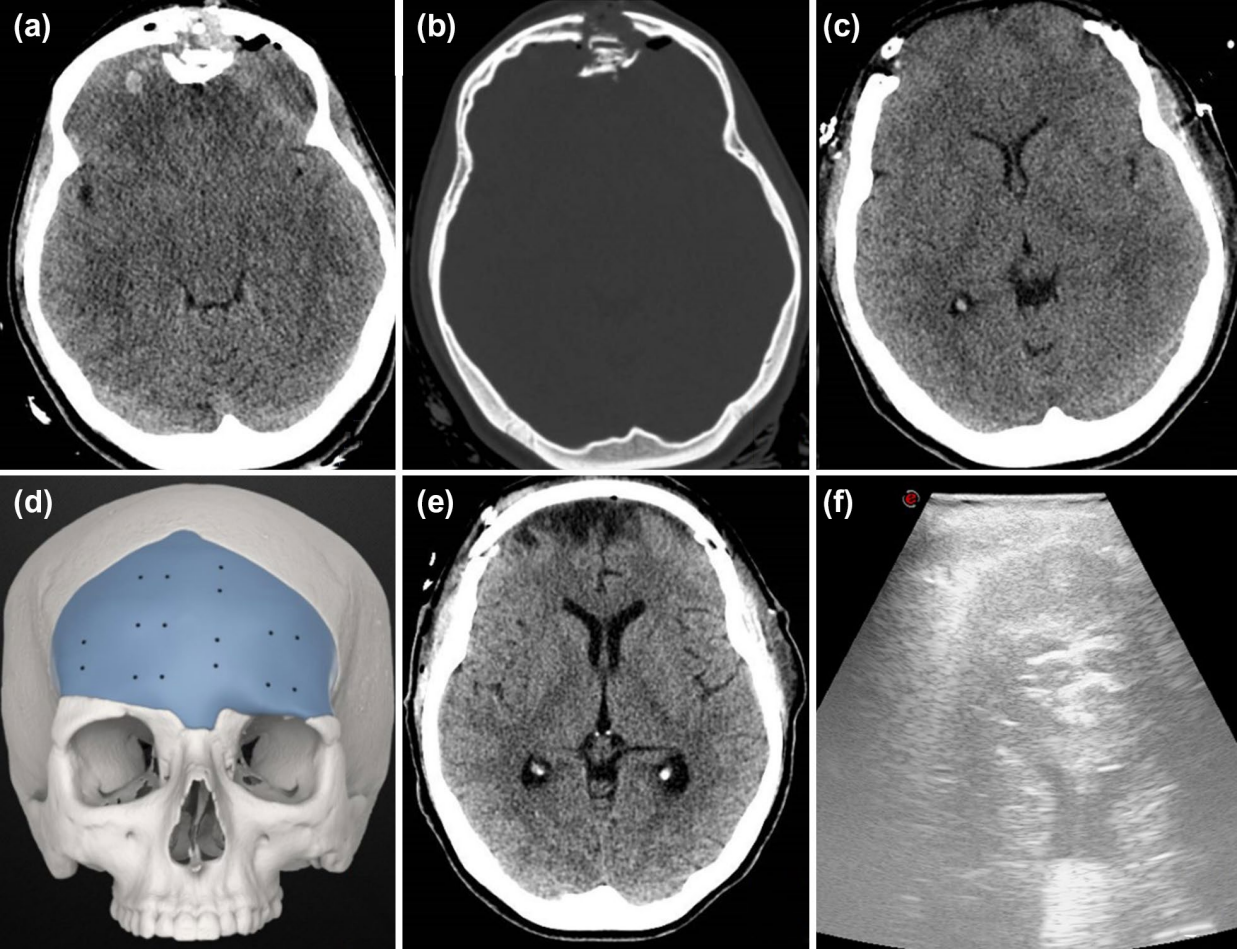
Preoperative CT scan showing anterior and posterior wall frontal sinus fracture along with bifrontal contusions (a, b) in a young patient who underwent bifrontal craniectomy with frontal sinus cranialization after TBI (c) and subsequent CP (d, e) in our hospital, not included in the present study. f, postcranioplasty ultrasound appearance of small ventricular size. CT, computed tomography, CP, cranioplasty, TBI, traumatic brain injury
